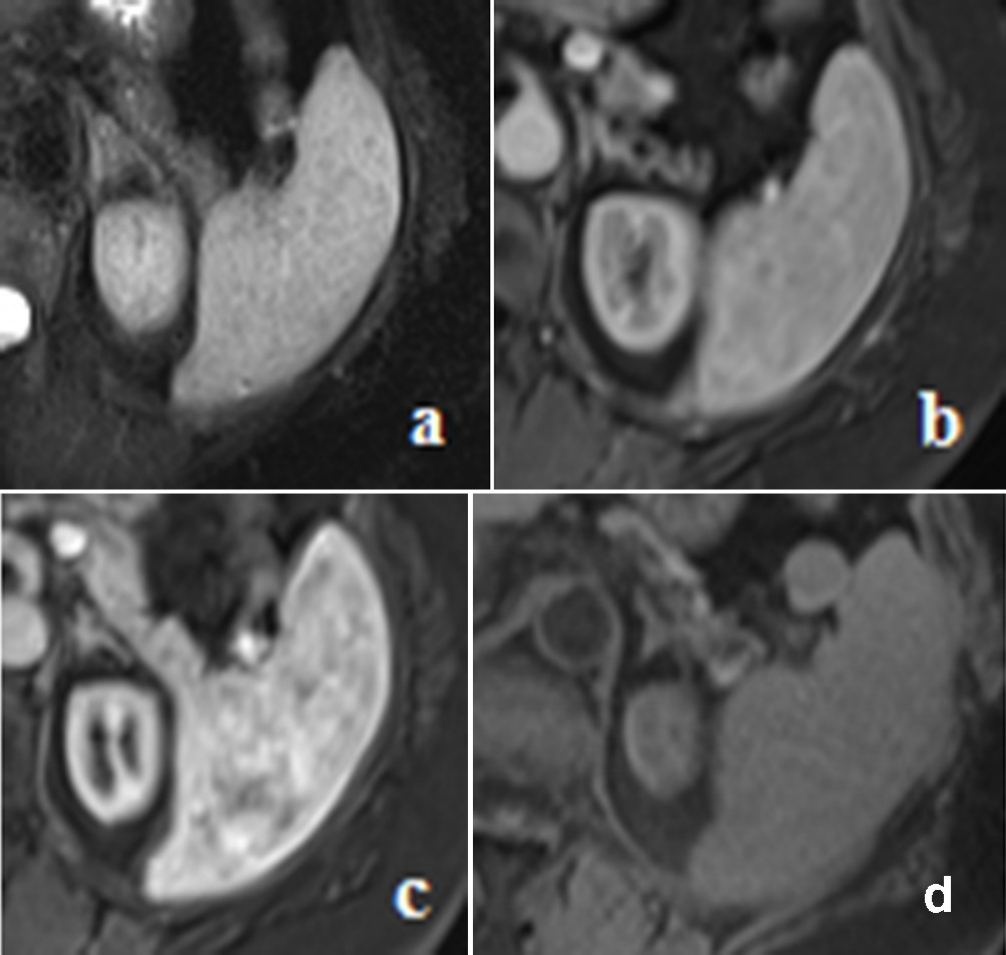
(from left to right): Abdominal MRI with paramagnetic contrast medium: no
